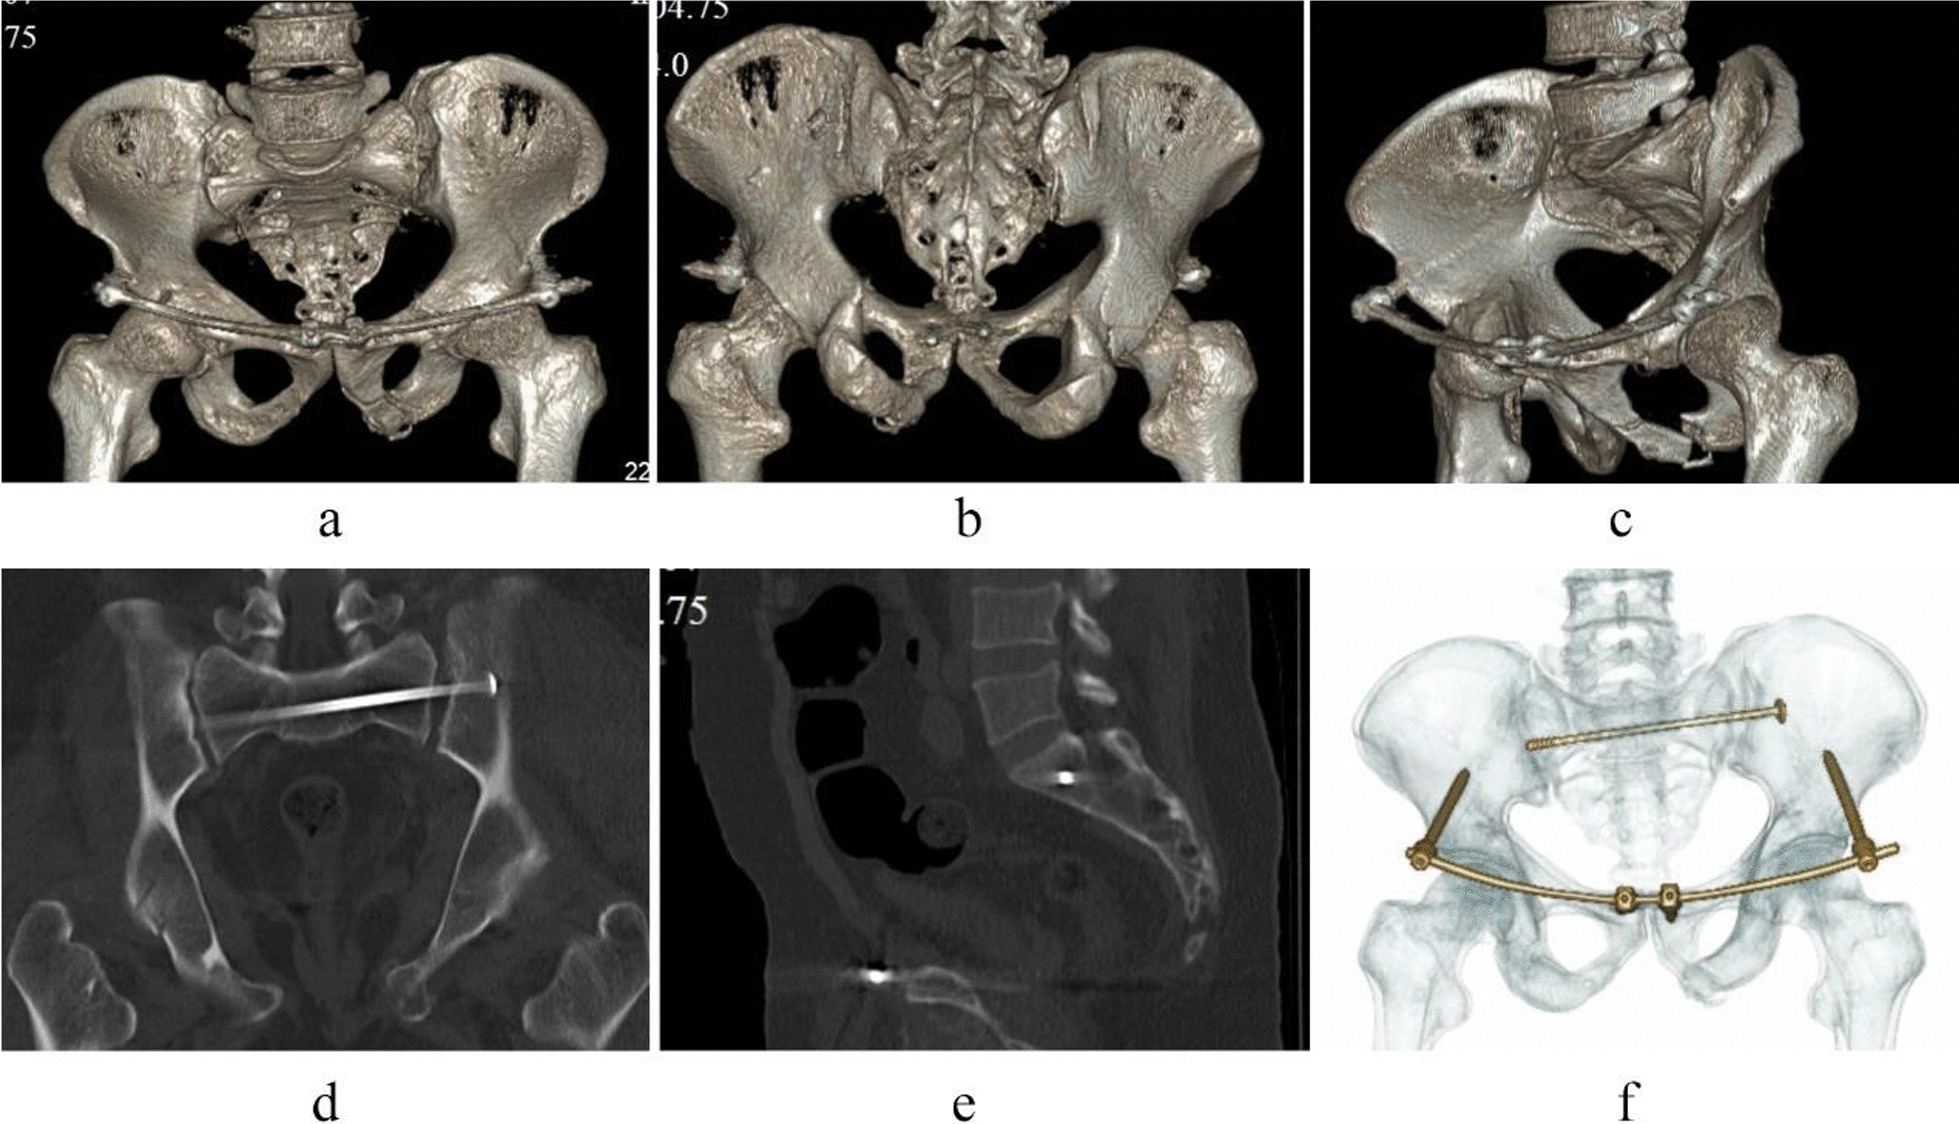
Postoperative CT images of case 3. (a–c, f CT 3D reconstruction image, d CT sagittal image, e CT coronal slice image.)
